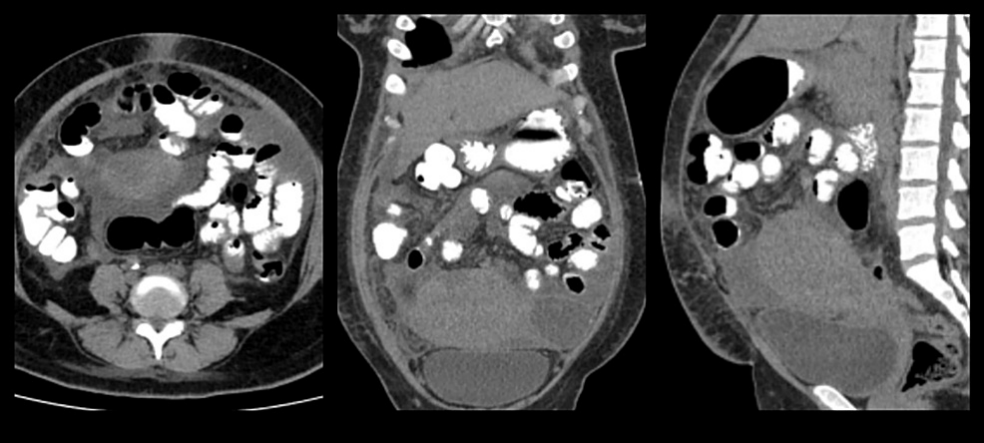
CT scan showing bulky postpartum uterus and moderate fluid collection with peritoneal thickening and enhancement with omental fat stranding.
